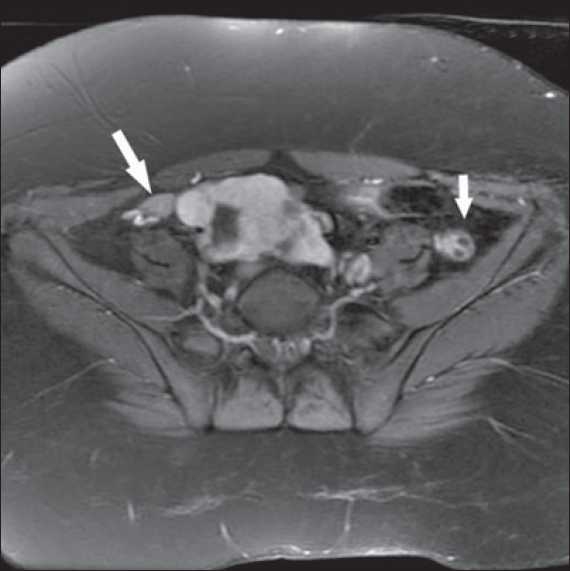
MRI showing bilateral ovaries
